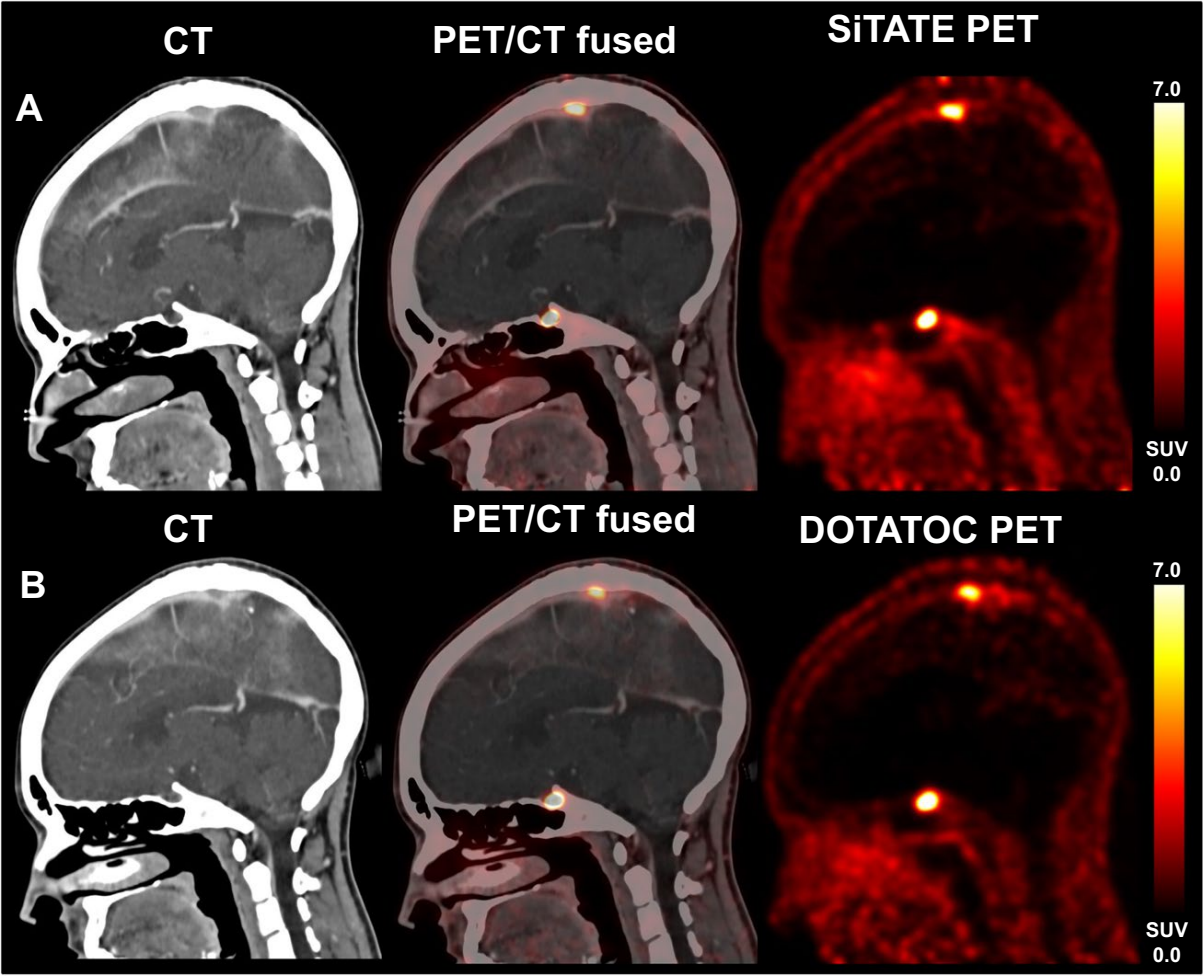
Follow-up imaging using [^18^F]SiTATE PET imaging: a patient with residual tumor remnant with extension to the superior sagittal sinus. The lesion at the superior sagittal sinus showed strong SSTR expression on [^18^F]SiTATE (A, SUV_max_ 8.6) without relevant changes in the course of 38 months without tumor-specific treatment. SSTR-expression on [^68^Ga]Ga-DOTATOC was comparable in the previous medical history (B, SUV_max_ 6.1)
